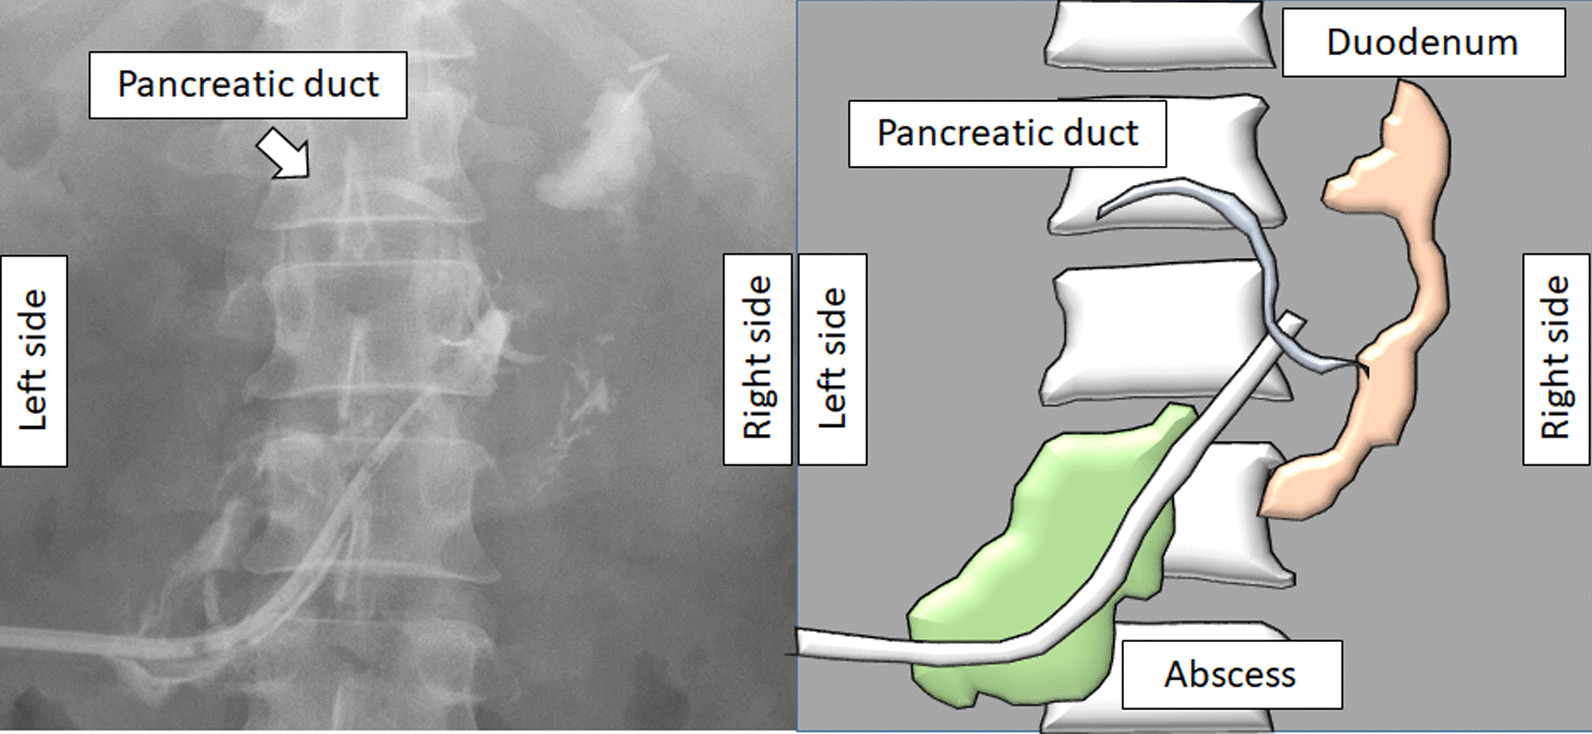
Contrast radiographic image through the percutaneous drain showing the distal part of the main pancreatic duct.
